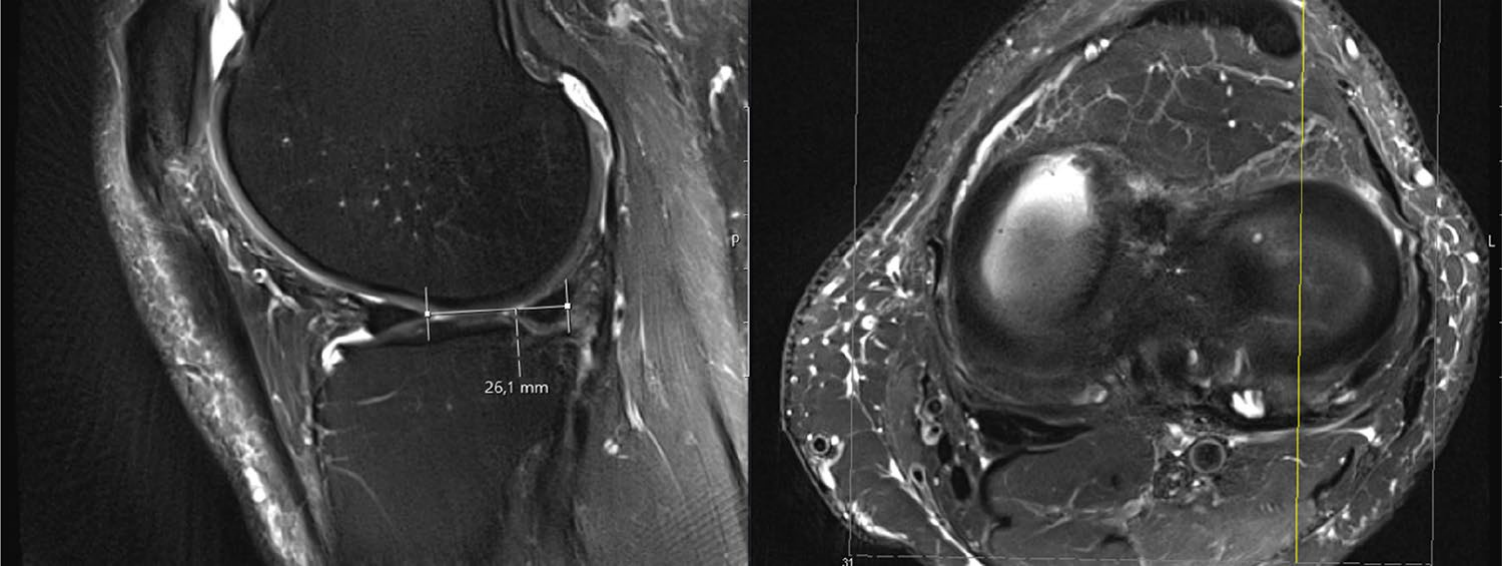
Magnetic resonance imaging. Measuring of the sagittal extrusion. The yellow line indicates the position of measuring. The distance between the inner margin of the lateral meniscus anterior horn and the posterior meniscocapsular junction was measured according to Ahn et al. [2]
